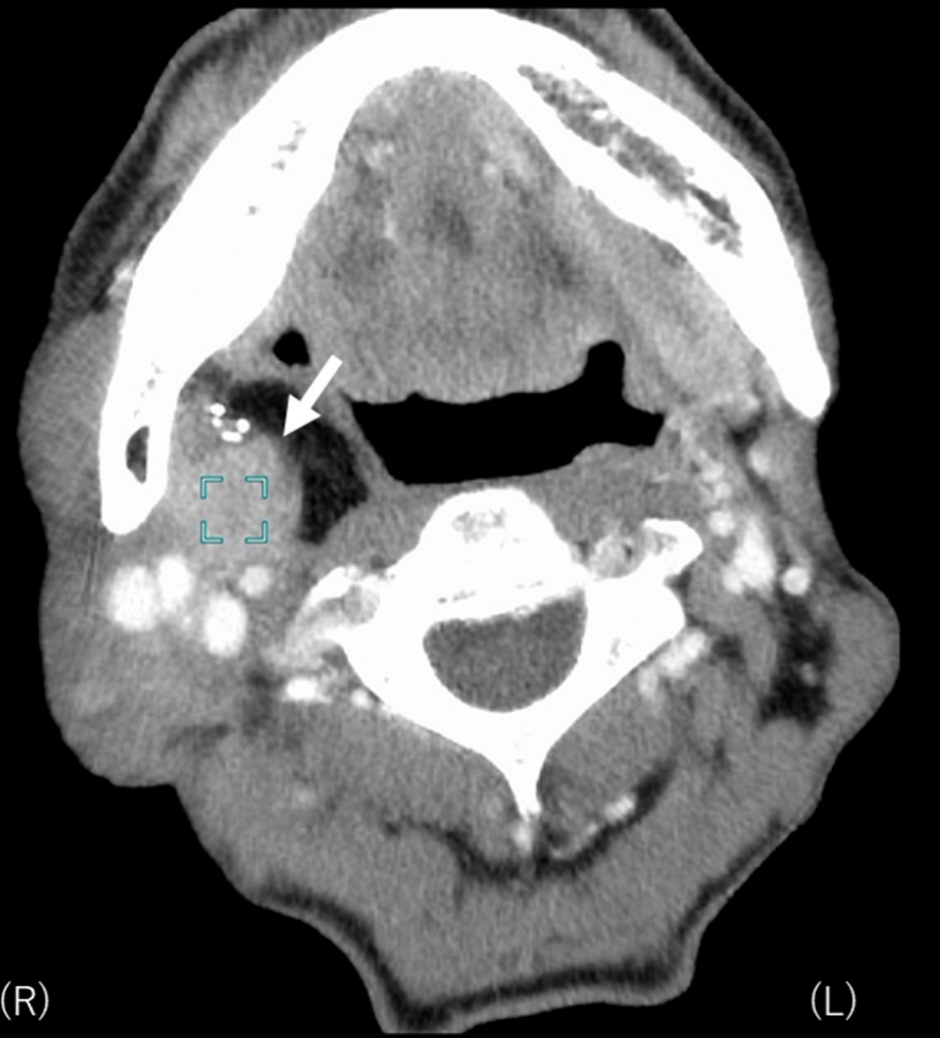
CT of the recurrent tumor beneath the ALT flap in the right side of the tonsillar area (white arrow) touching the right internal carotid artery. ALT, anterolateral thigh.
